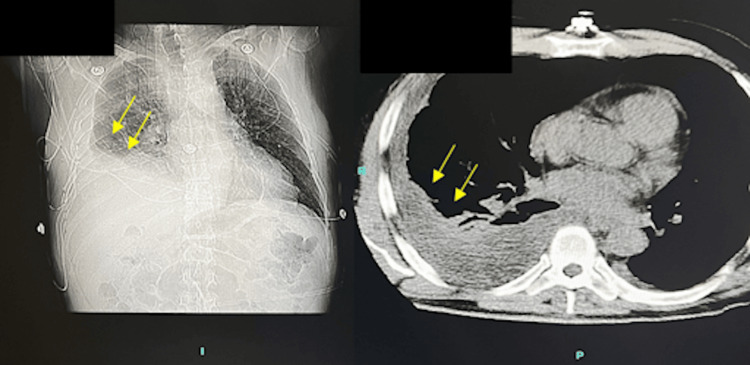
CT scan showing persistent right pleural effusion following administration of antimicrobials CT, computed tomography
